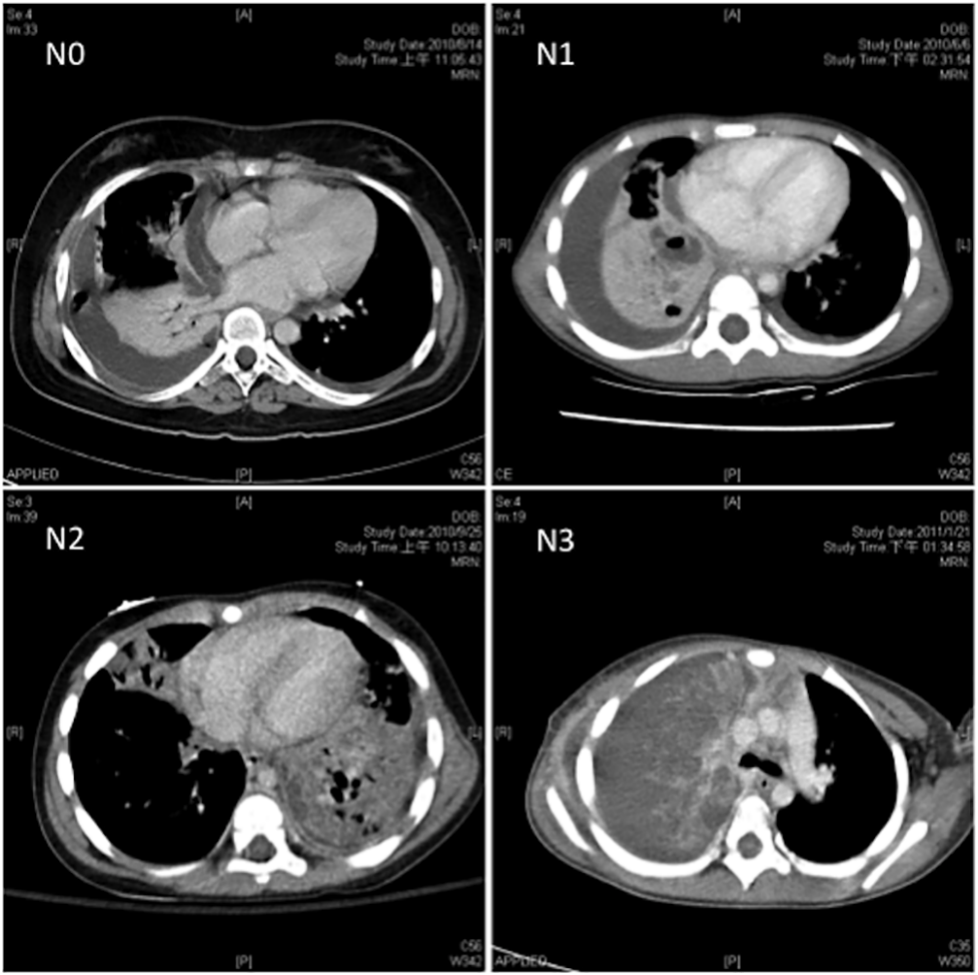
Computed tomographic grading of pneumonia with necrotizing changes. Severity of lung necrosis was measured on the basis of the absence of contrast uptake in computed tomography. Necrotic areas that were 0%, less than 30%, between 30 and 80%, and more than 80% were further categorized into no (N0), mild (N1), moderate (N2), and massive (N3) necrosis.
